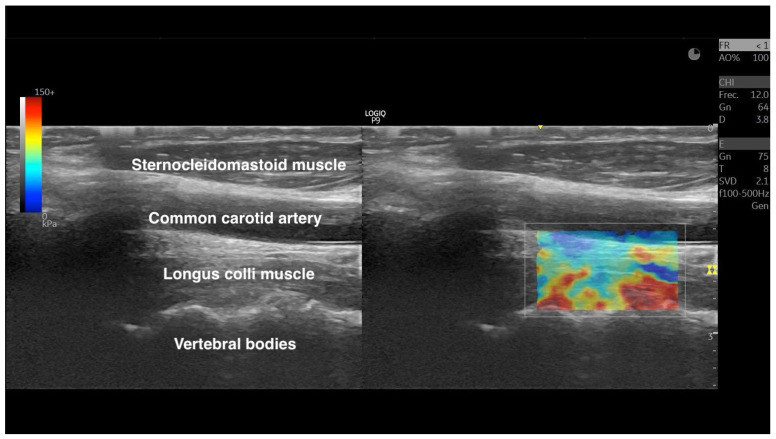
Representative ultrasound of the longus colli. The left panel shows the B-mode image, and the right panel overlays shear-wave elastography, with a color-coded ROI depicting the tissue stiffness distribution. The color map represents the kPa scores; cooler colors indicate lower stiffness and warmer colors indicate higher stiffness, according to the displayed quantitative scale.
